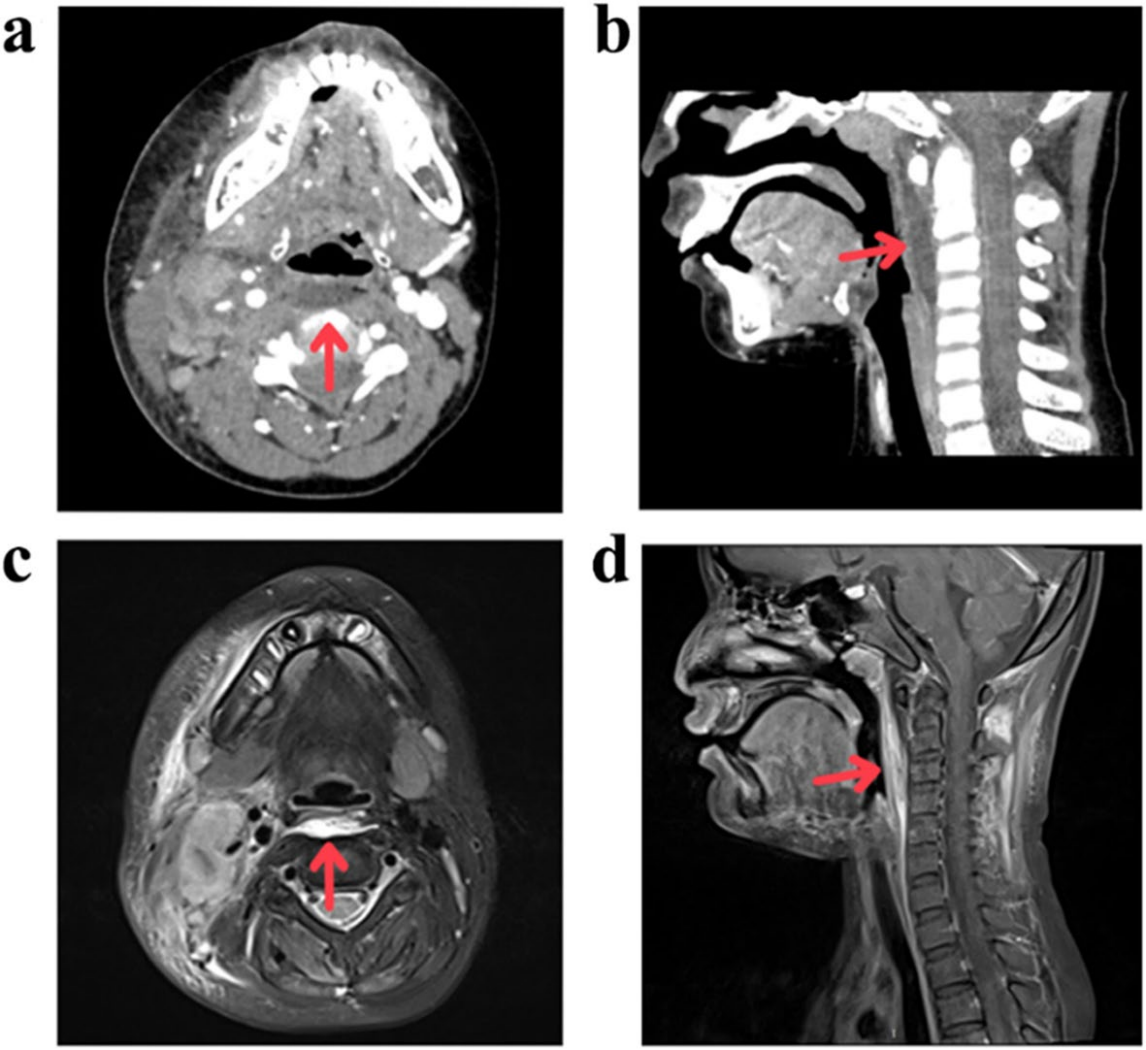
Neck radiological findings of KD initially presenting as retropharyngeal abnormality. a, b Axial and sagittal enhanced neck CT images showing a widening of the retropharyngeal space with a hypodense area without significant ring enhancement (arrow). c, d Axial T2fs MR image and sagittal postGd T1fs MR image showing a retropharyngeal fluid collection with soft tissue oedema without significant ring enhancement (arrow)
